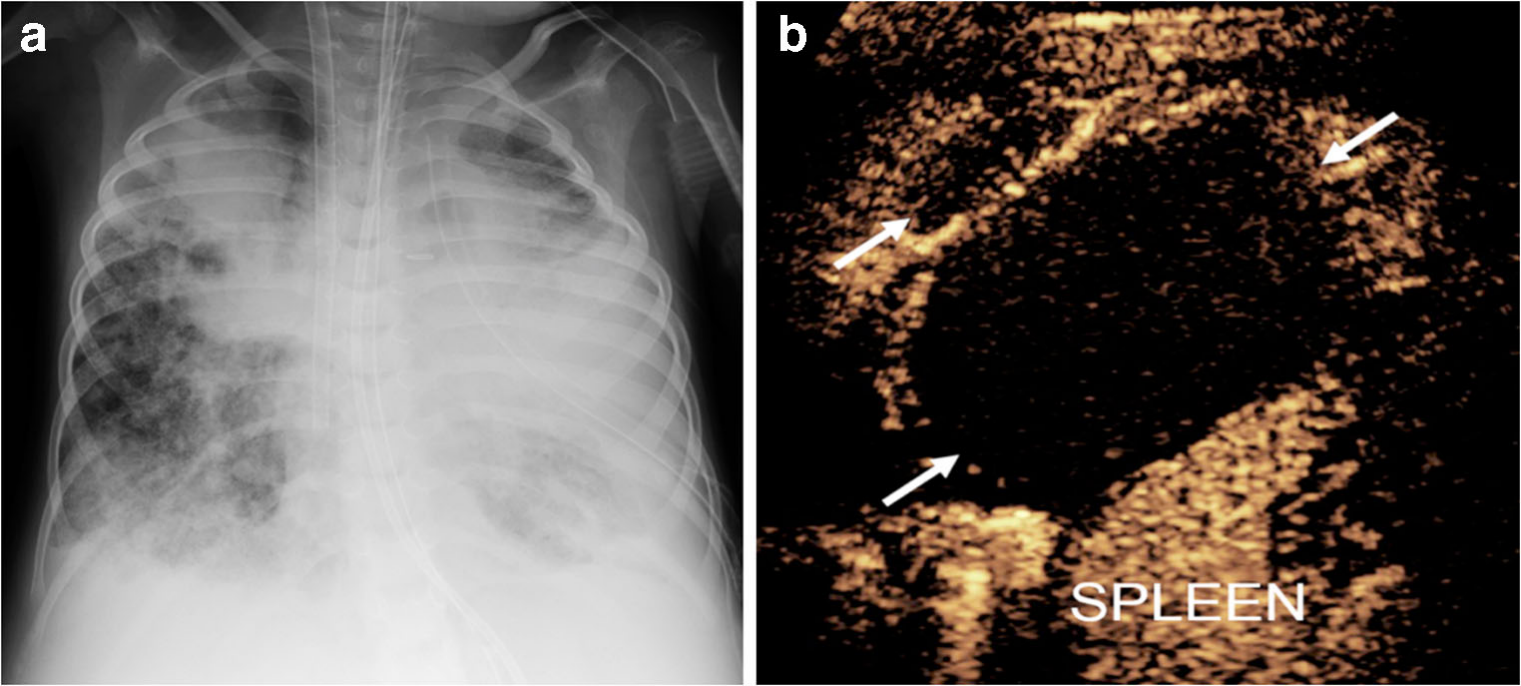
Lung infection and concern for lung abscess in a 6-year-old boy with chronic renal and respiratory failure caused by prune belly syndrome, on extracorporeal life support. a Posteroanterior chest radiograph demonstrates extensive multifocal opacities throughout both lungs, more confluent in the right upper lobe and left mid and lower lung lobes. b Contrast-enhanced ultrasound (CEUS), coronal view of the left lung. Following intravenous administration of contrast agent, there is a large non-enhancing area (*arrows*) within the left lower lobe with a smaller similar non-enhancing area anteriorly. These findings are in keeping with necrotizing pneumonia
